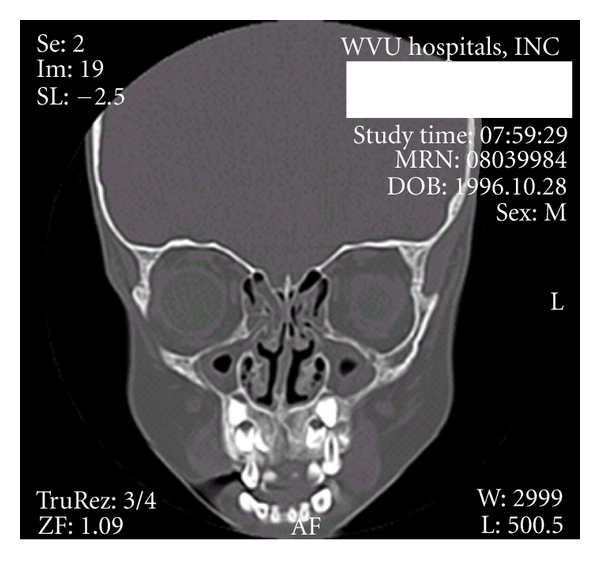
Coronal CT scan of the sinuses demonstrating significant disease in both ethmoids and maxillary sinuses with blockage of the OMC.
